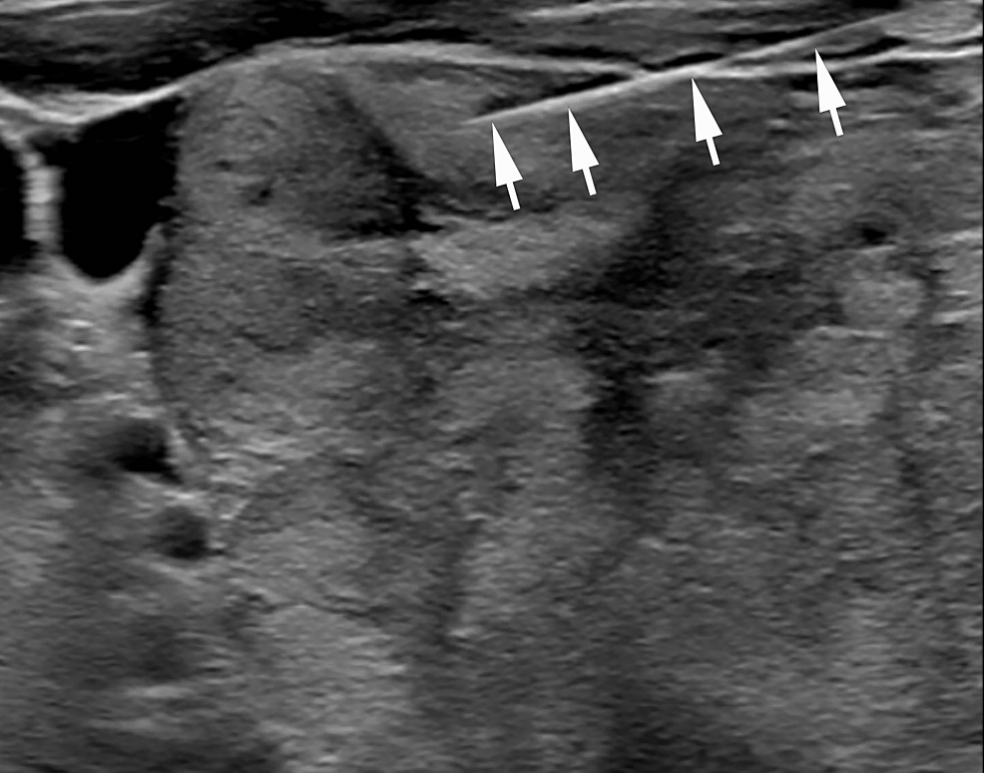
Transverse view of the ultrasound-guided fine needle aspiration of the left thyroid lobe. The arrows are pointing to the fine needle.
